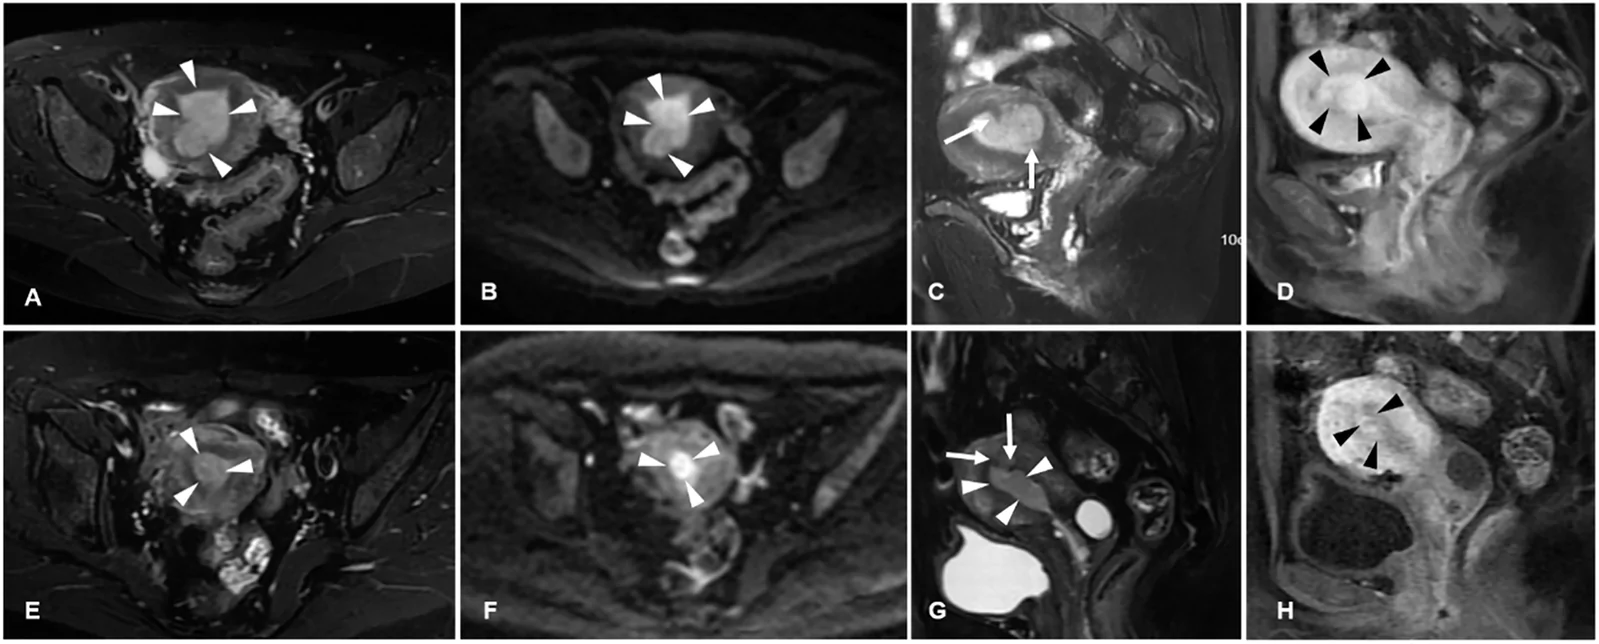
LG-ESS from pelvic magnetic resonance images of a 50-year-old (A–D) woman and a 55-year-old (E–H) woman. Axial T2W (A) and DW (B) images showing a poorly defined mass extending from the endometrial cavity into the myometrium, predominantly located within the cavity, appearing hyperintense on T2WI and DWI (arrowheads). Sagittal T2W image (C) showing disrupted myometrial bundles angling toward the endometrial cavity (arrows). Sagittal T1-weighted contrast-enhanced image (D) showing marked enhancement of the lesion (arrowheads). Axial T2W (E) and DW (F) images showing an irregular mass within the endometrial cavity, which appears hyperintense (arrowheads). Sagittal T2-WI (G) showing disrupted myometrial bundles angling toward the endometrial cavity (arrows). Sagittal T1-weighted contrast-enhanced image (H) showing marked enhancement of the lesion (arrowheads)
